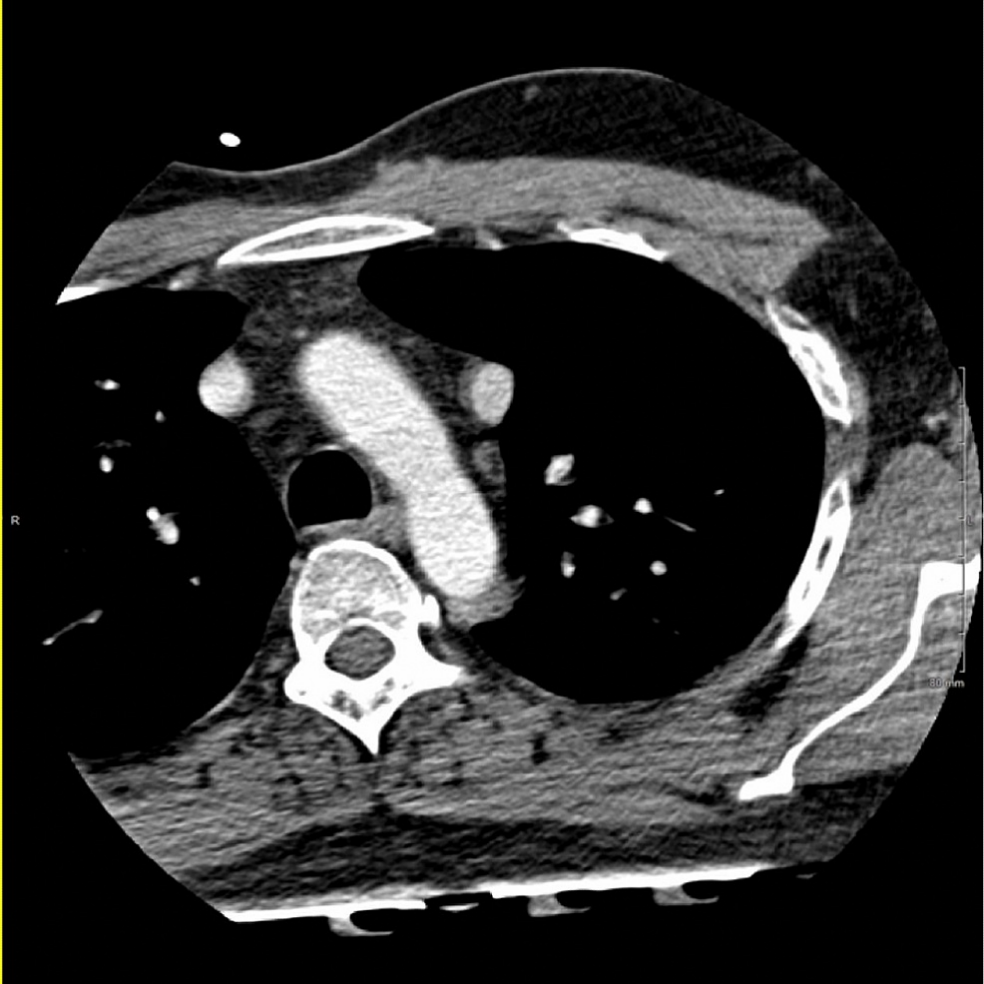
Axial CT image at the level of the aortic arch demonstrates a right SVC and a left SVC. SVC: superior vena cava.
